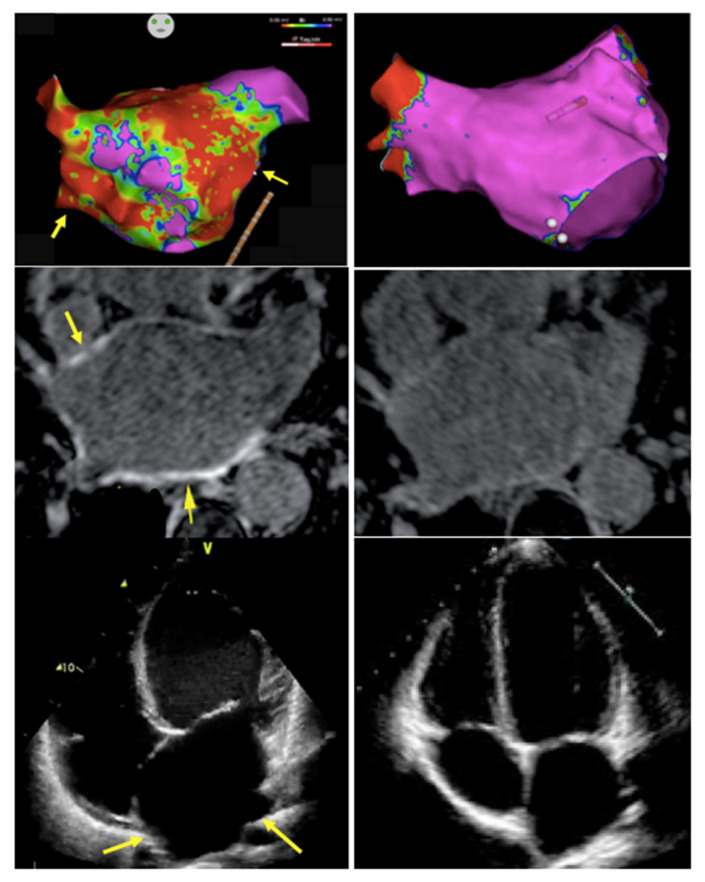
Substrate Severity: Multimodality assessment of the underlying atrial substrate to guide individualized treatment strategies. Figure 2 demonstrates three examples of severely diseased left atria compared to structurally normal ones. (Top row): Electro-anatomical voltage map of the left atrium: (Left) Left atrium with extensive scarring (red areas corresponding to bipolar voltage <0.05 mV, indicating scarring); (Right) Left atrium with normal “healthy” bipolar Voltage >0.5 mV (pink areas). (Middle row) Atrial CMR images: (Left) late gadolinium enhancement (yellow arrows) within the anterior and posterior wall of the left atrium indicating fibrosis; (Right) no detectable LGE. (Bottom row*)* TTE Images Apical 4-chamber view: (Left) significantly enlarged left atrium; (Right) normal-sized left atrium.
